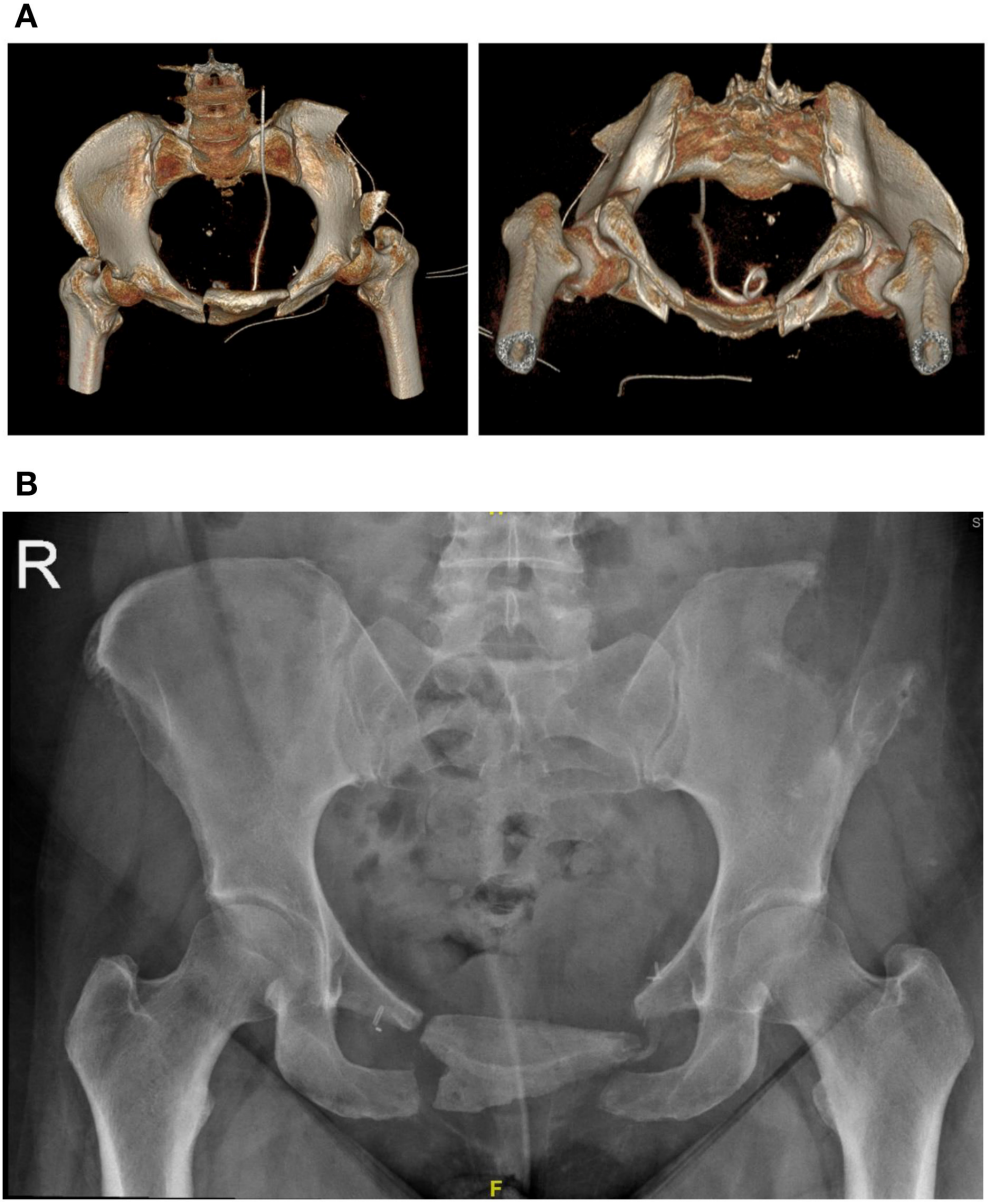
(A) day 1 post surgery. 3D reconstructions of a CT pelvis demonstrating the iliac crest graft *in-situ*. (B) 6-month follow up X-Ray demonstrating stable positioning of the graft.
